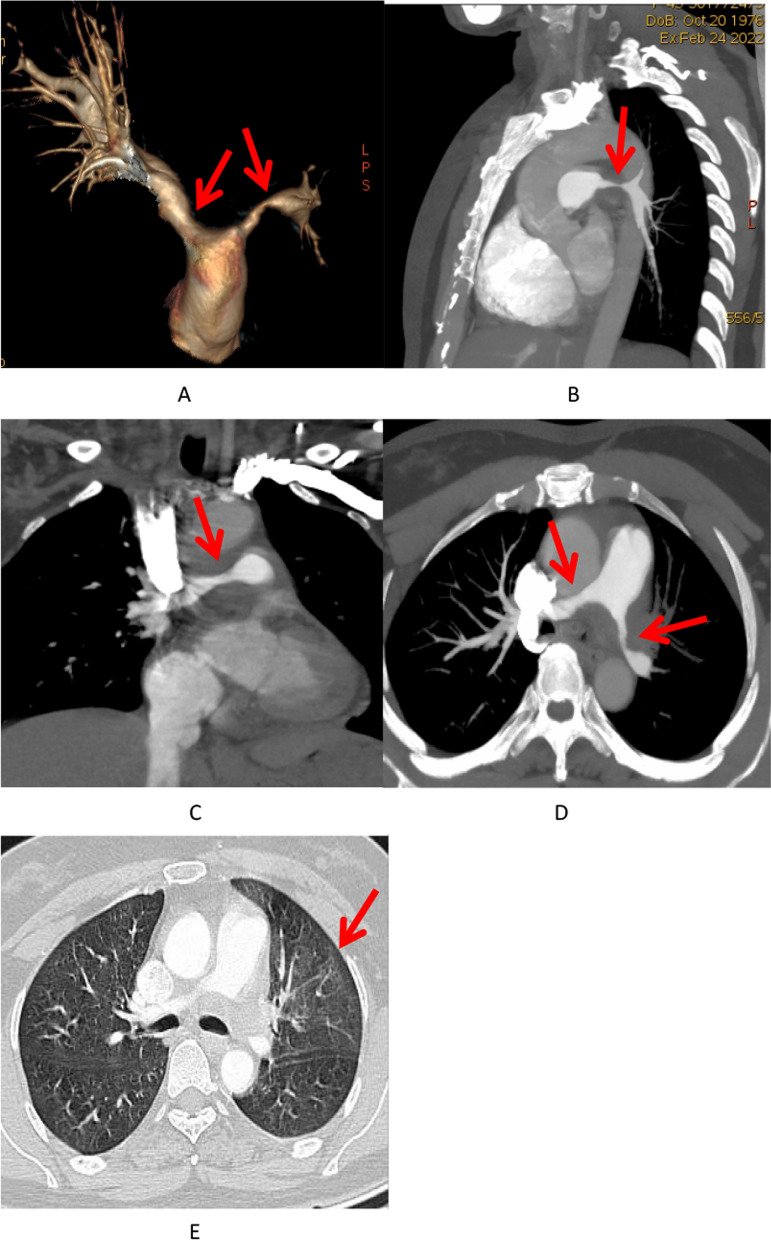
A–D Pulmonary computed tomography angiography (CTA) images. E HRCT. A, D showing thick walls of both main PA and stenosis of both main PA lumen (red arrows). B Display severe stenosis of the left PA (red arrows). C Showing mild-to-moderate stenosis of the right PA (red arrows). (E) Display the mosaic sign of the left lung (red arrows)
